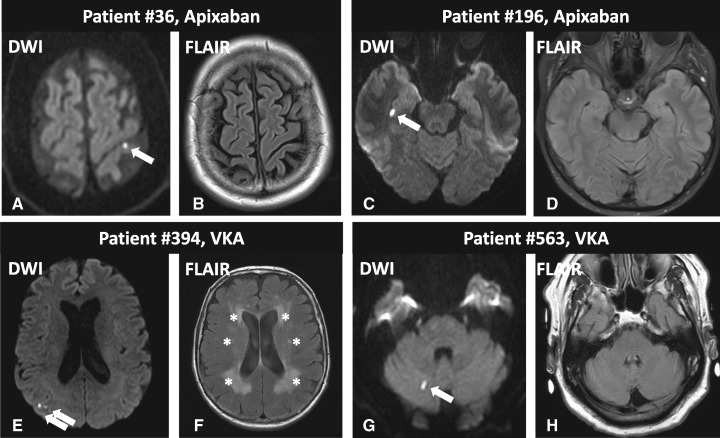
Examples of acute brain lesions detected in the brain magnetic resonance imaging sub-study. Acute brain lesions (arrows in *A*, *C*, *E*, *G*) are found by high-resolution diffusion-weighted brain magnetic resonance imaging without corresponding lesions in the fluid-attenuated inversion recovery images (arrows in *B*, *D*, *F*, *H*). Shown are representative lesions in two patients randomized to apixaban (*A*/*B*; *C*/*D*) and in two patients randomized to vitamin K antagonist (*E*/*F*; *G*/*H*). The fluid-attenuated inversion recovery images also detected chronic white matter lesions [asterisks (*) in F].
